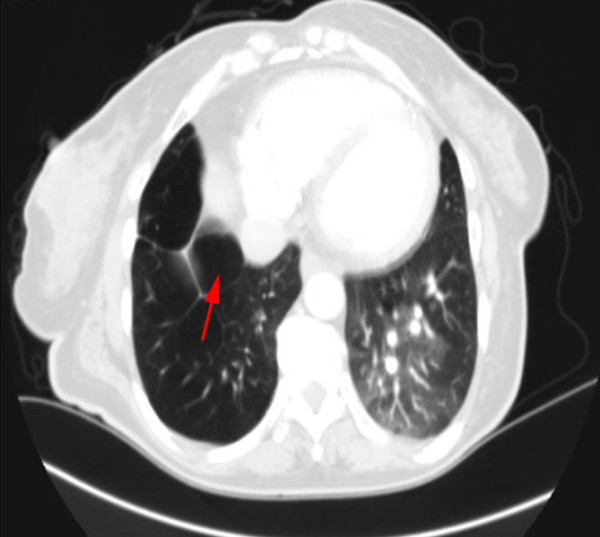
Chest high resolution computed tomography scan demonstrating hyperinflation (air trapping) in the pulmonary parenchyma (red arrow).
